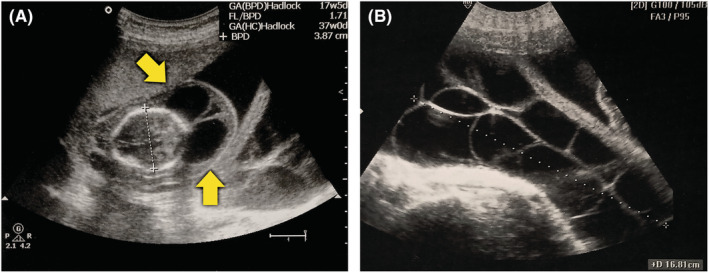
Transabdominal ultrasound examination. (A) Axial view of the fetus showing a cephalic cystic hygroma with two oval‐shaped fluid chambers that lie between the skull and the scalp (arrows). The biparietal diameter equals 3.87 cm. (B) Transverse suprapubic view of the mother showing multiple cystic formations in the right ovary with regular walls and anechoic content. The maximal diameter of the complex equals about 17 cm.
